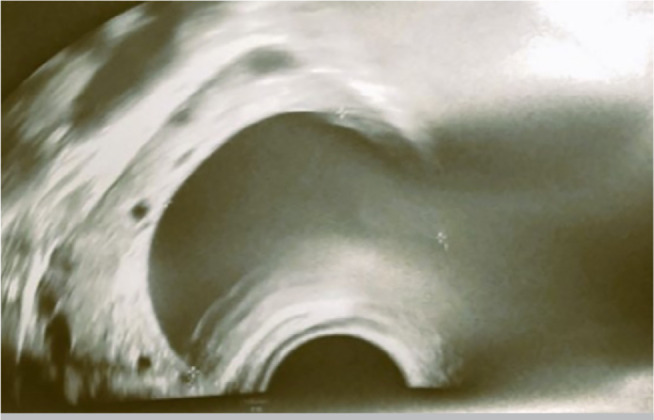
Ultrasound scan showing edema and cyst in the RT ovary.
